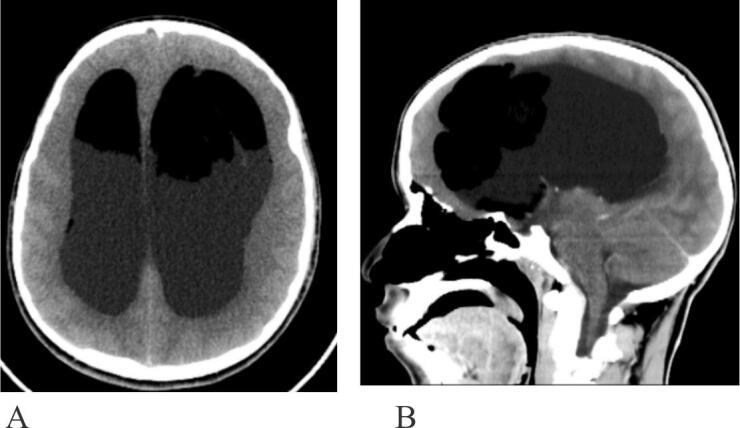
Axial CT without contrast (A) and sagittal CT with contrast (B) show fat attenuation of the lesion and associated hydrocephalus.
